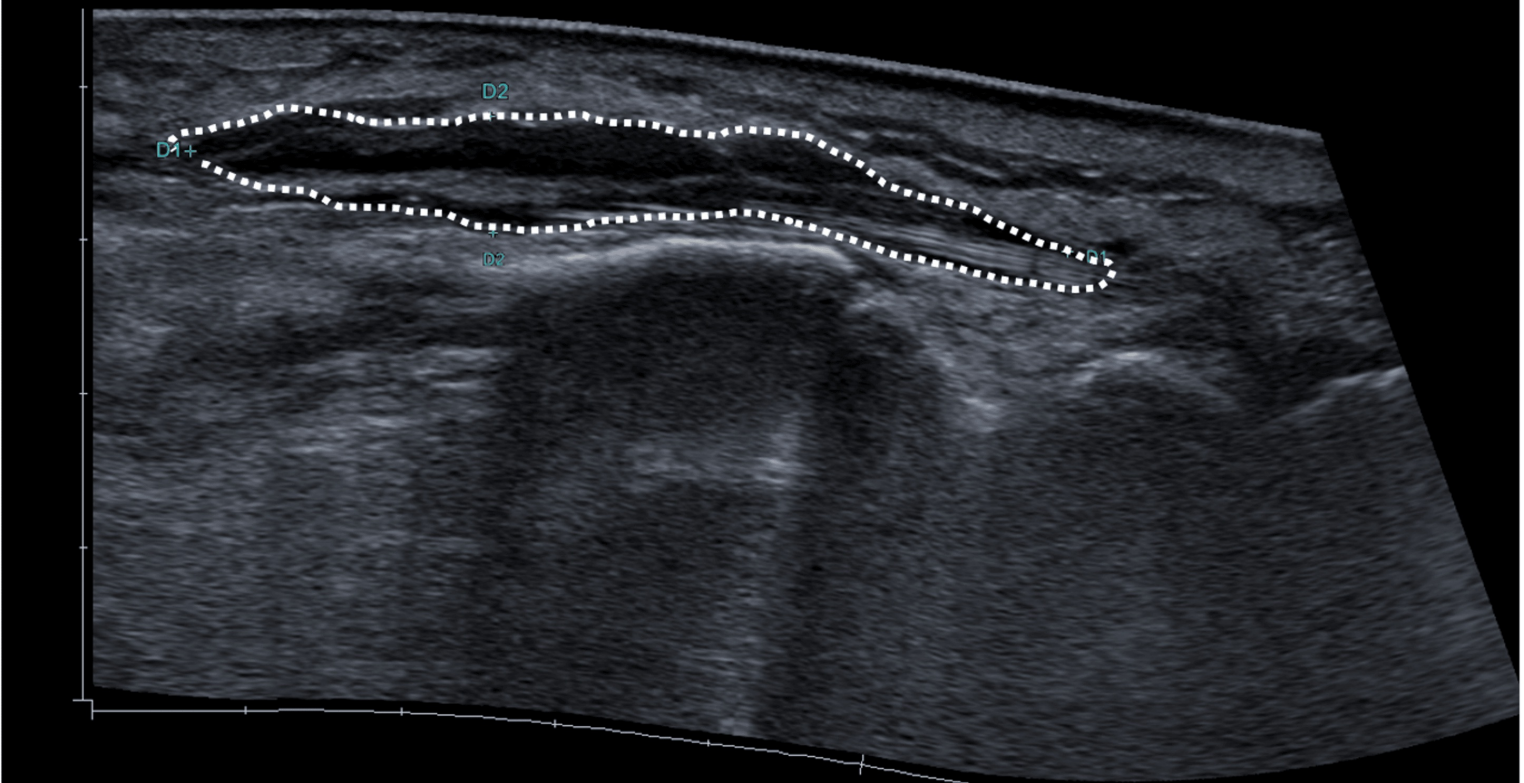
Ultrasound of soft tissues. Significant edema of the subcutaneous tissue in the described area is observed (white lines). Additionally, a hypoechoic, irregularly bordered, avascular lesion is visualized on color Doppler, corresponding to a collection measuring 57 × 7 × 51 mm, with a calculated volume of 11 mL.
